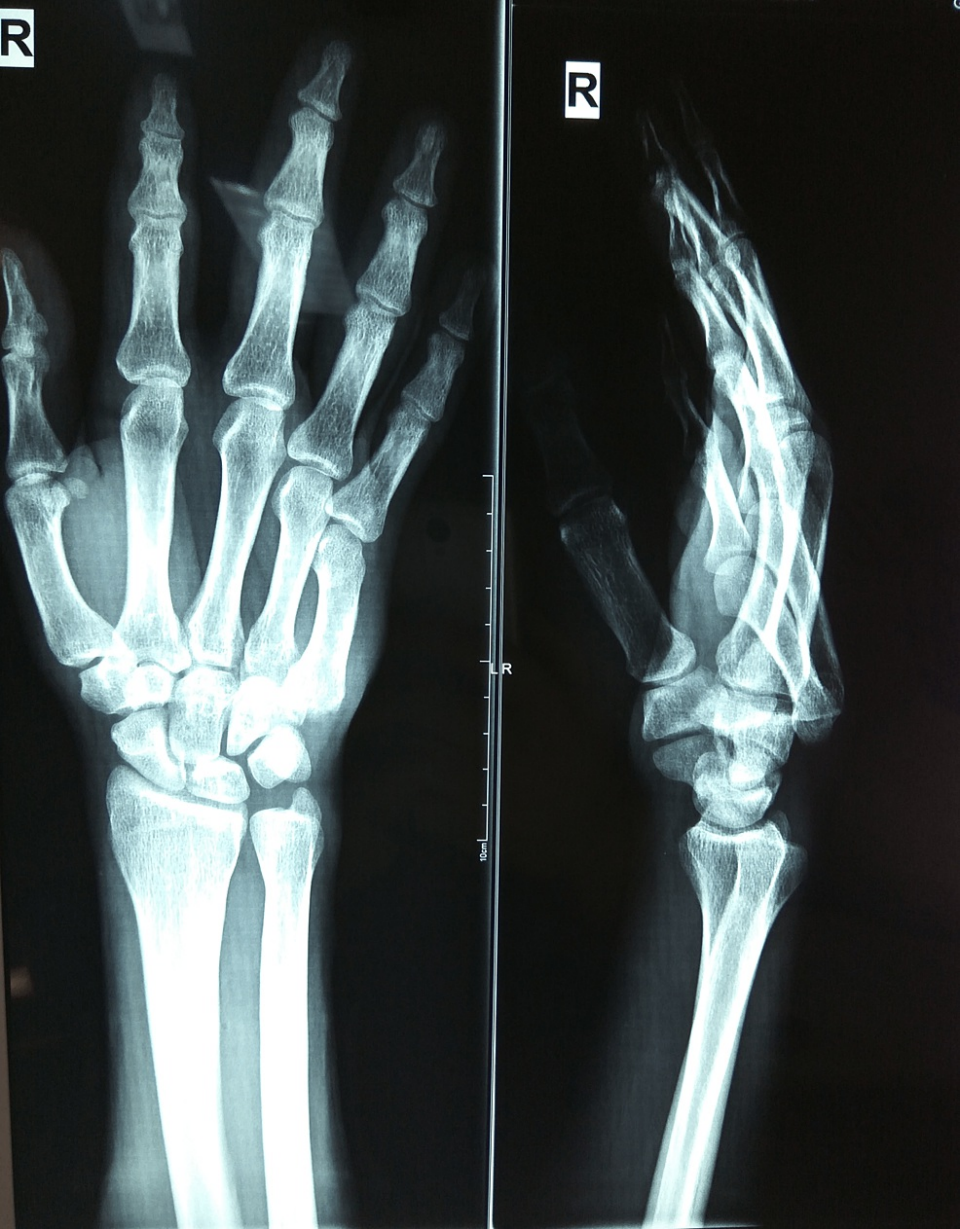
X-ray of the injured hand The anteroposterior and lateral views depict the isolated dorsal dislocations of both the fourth and fifth carpometacarpal joints
